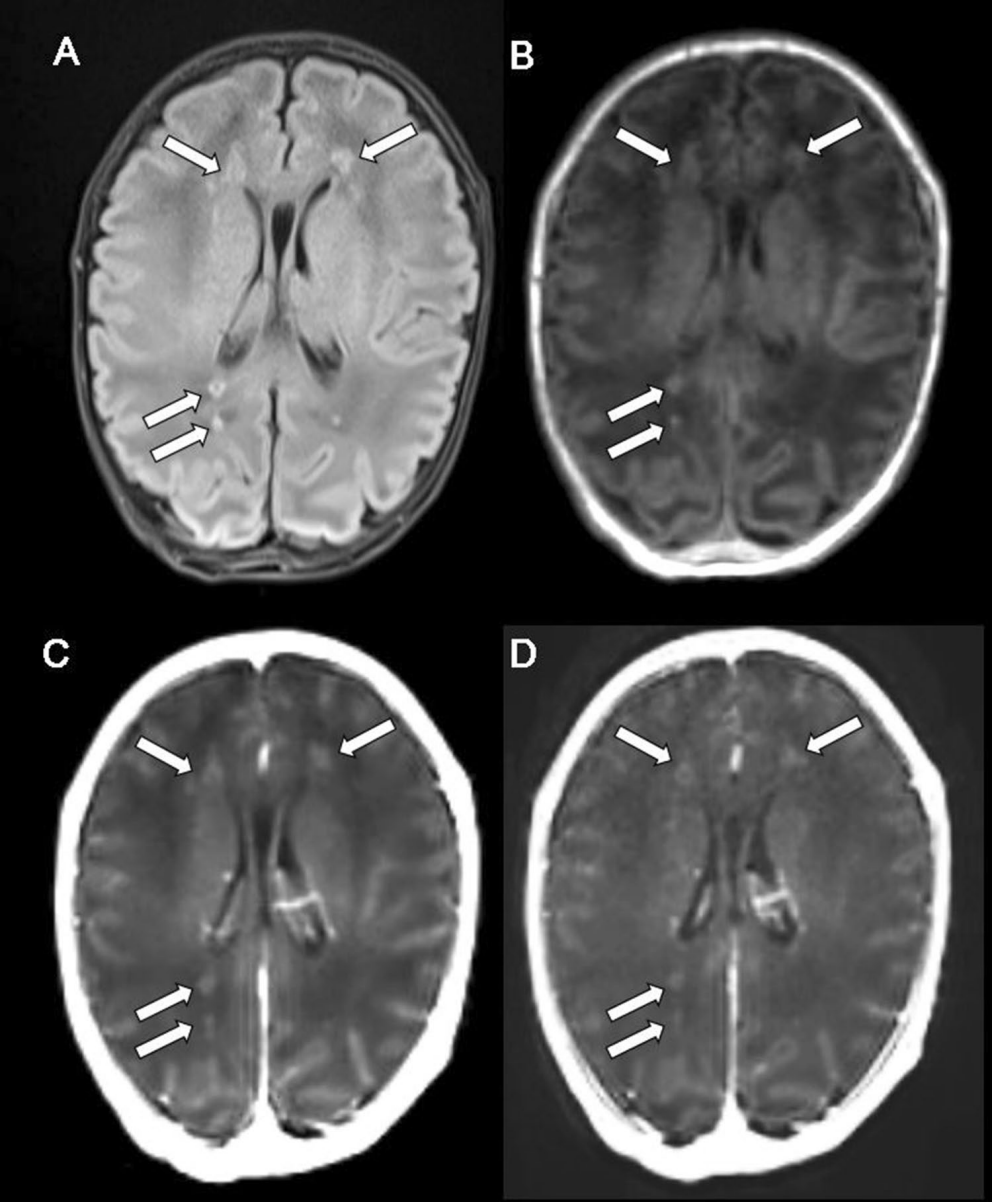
Periventricular leukomalacia on conventional and wave-CAIPI MPRAGE. A 1-month preterm neonate (gestational age: 36 weeks) undergoes an MRI for the evaluation of brain parenchymal lesions. Multifocal FLAIR (A) and precontrast T1 MPRAGE (B) high signal intensity lesions without enhancement on both conventional MPRAGE (C) and wave-CAIPI MPRAGE (D) which suggest areas of hemorrhage are discovered in both periventricular white matter. The patient is diagnosed with periventricular leukomalacia.
